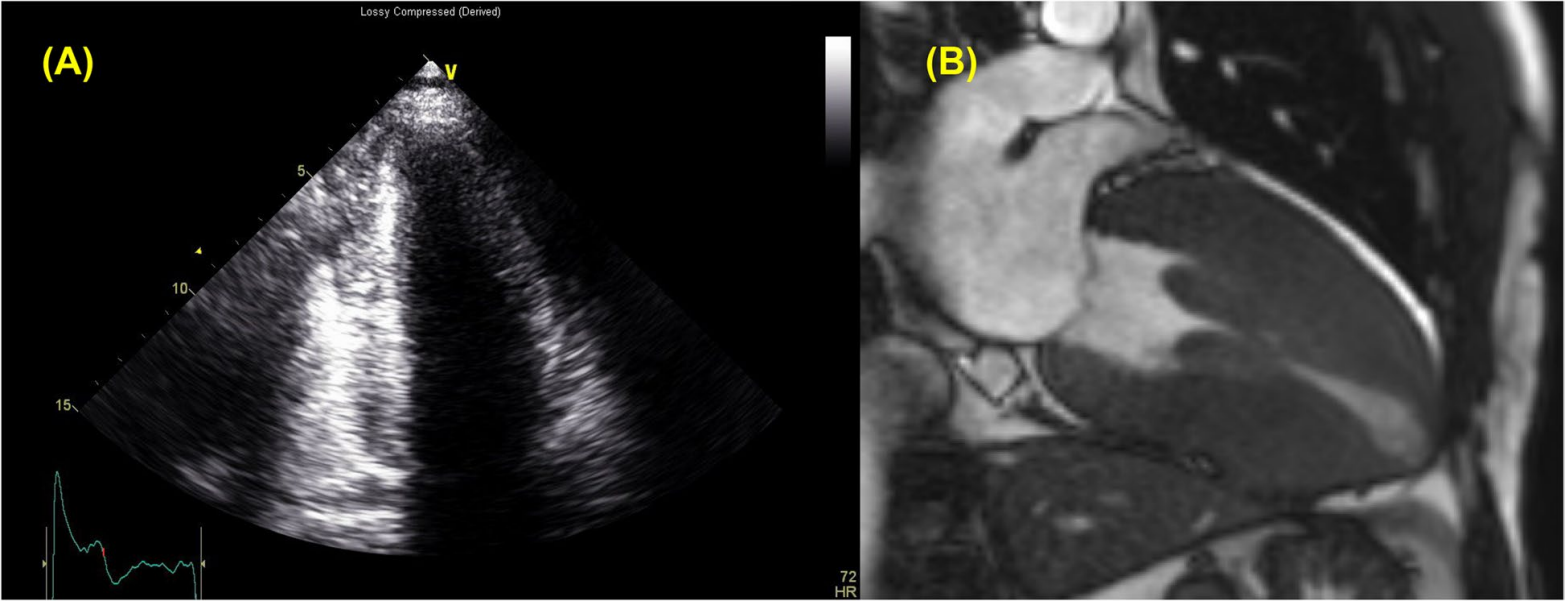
Patients with apical aneurysm. Compared to the suboptimal image quality of (A) transthoracic echocardiography, B cardiac magnetic resonance imaging clearly reveals a small apical aneurysm during the systolic phase
